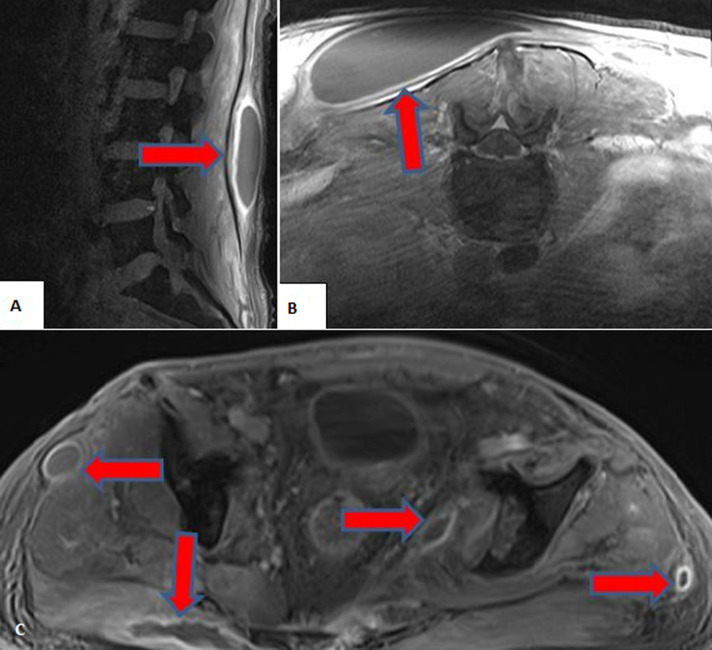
A) magnetic resonance imaging sections displaying; B) subcutaneous abscess in the left lumbar area; C) multiple abscesses (red arrows)
